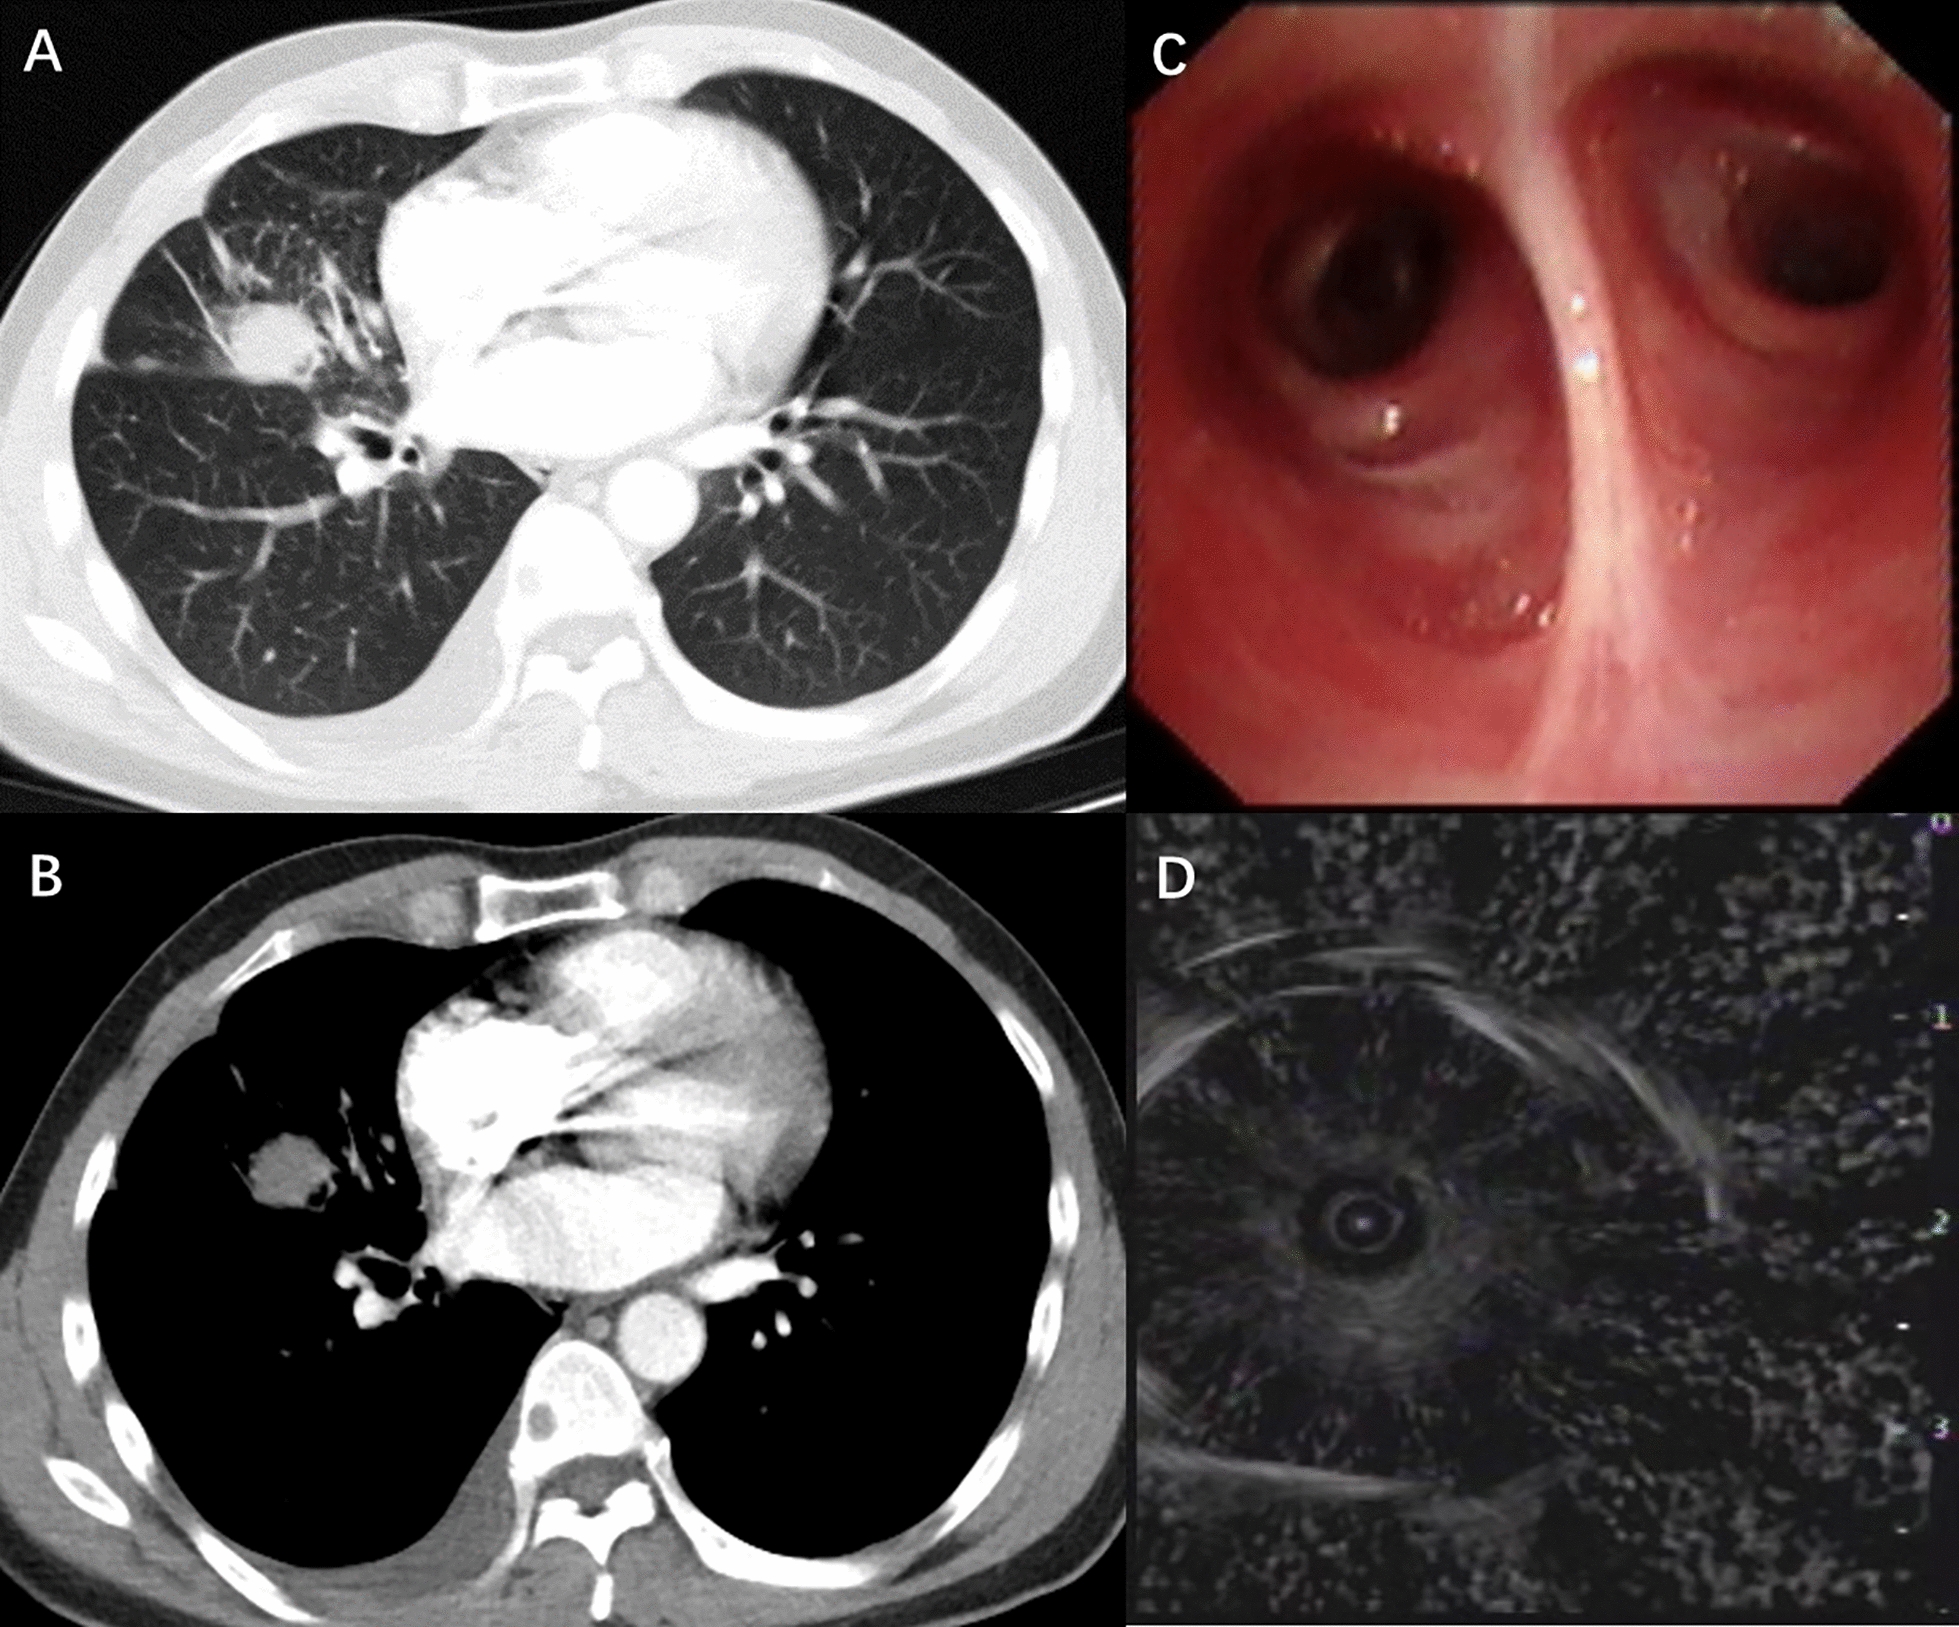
Case 2, a 42-year-old man who was diagnosed with non-small-cell lung cancer in the middle lobe of the right lung. A, B Chest CT, showing lesions in the lung window and mediastinal window. C, D Ultrasound from EBUS-GS, showing lesions around the ultrasound probe
